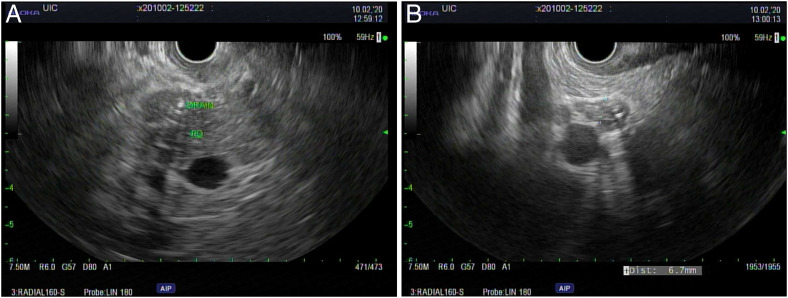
Endoscopic ultrasonography visualization of the patient's external-internal biliary drain (A) and dilated common bile duct (B). The common bile duct is dilated to a diameter of 7 mm and demonstrates a region of hypoechogenicity surrounding the drain, suggestive of bile sludge.
